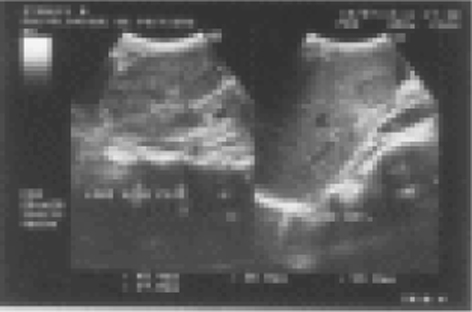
Transabdominal ultrasonography of liver showing multinodular HCC in left lobe and a nodular HCC with posterior enhancement in right lobe. (with permission) [19].
